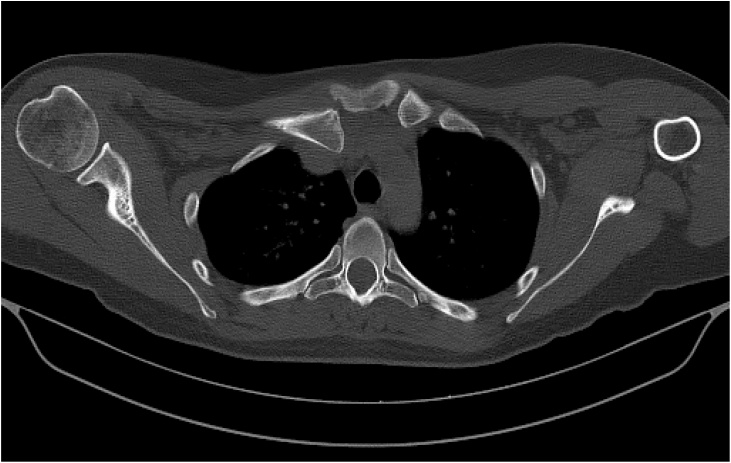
Axial CT without contrast at the level of the joint revealed a Salter Harris 2 fracture with posterior displacement at the right sternoclavicular joint. There was a small fragment of bone at the superior-anterior aspect of the joint represents the ossifying epiphysis. The relationship of the medial clavicle to the subclavian vein and brachiocephalic vein may is seen by subsequent CT with intravenous contrast.
